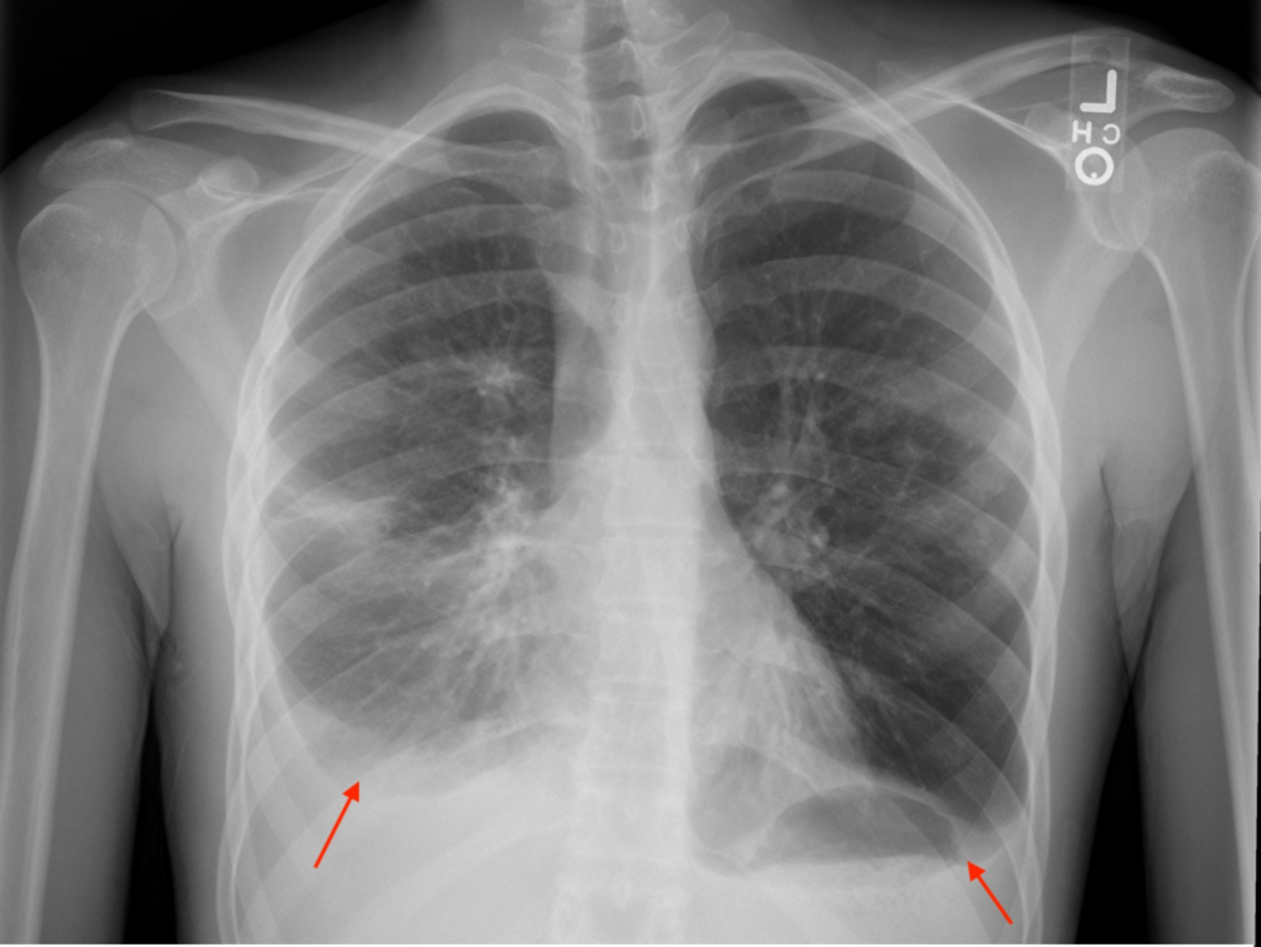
Chest x-ray with small to moderate size right pleural effusion (red arrow) and questionable opacities in the right middle lobe and anterior segment of the right upper lobe. Also note small left pleural effusion (red arrow).
